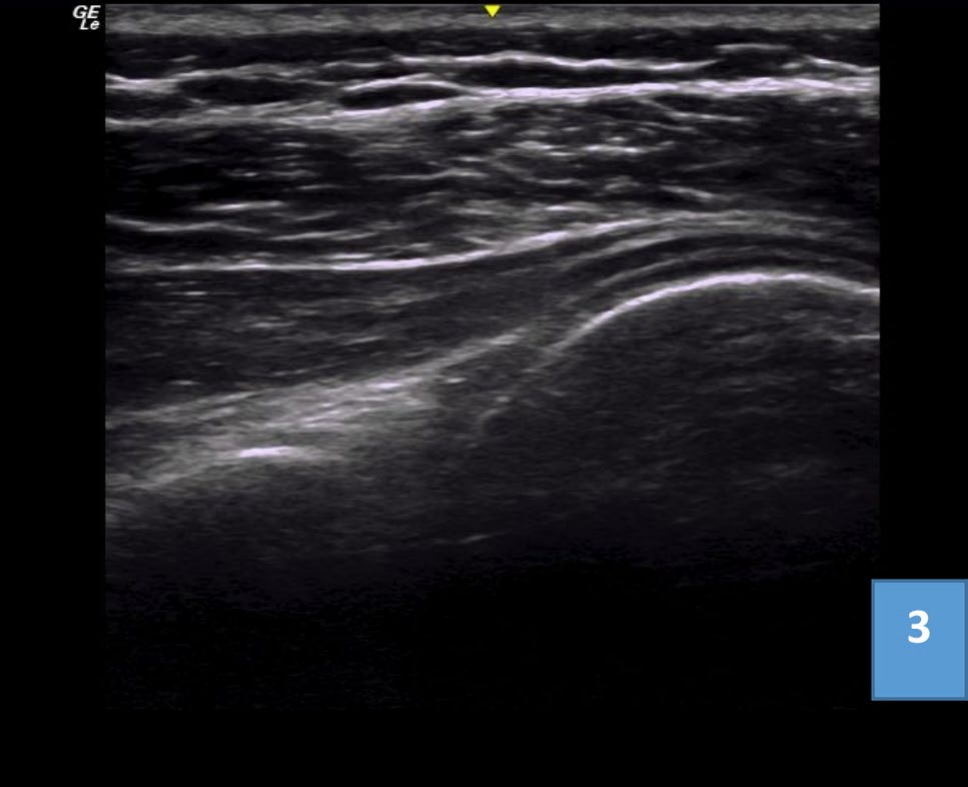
Posterior ultra-sonographic scan of shoulder showing posterior portion of head of humerus, infraspinatus muscle, and posterior joint recess
